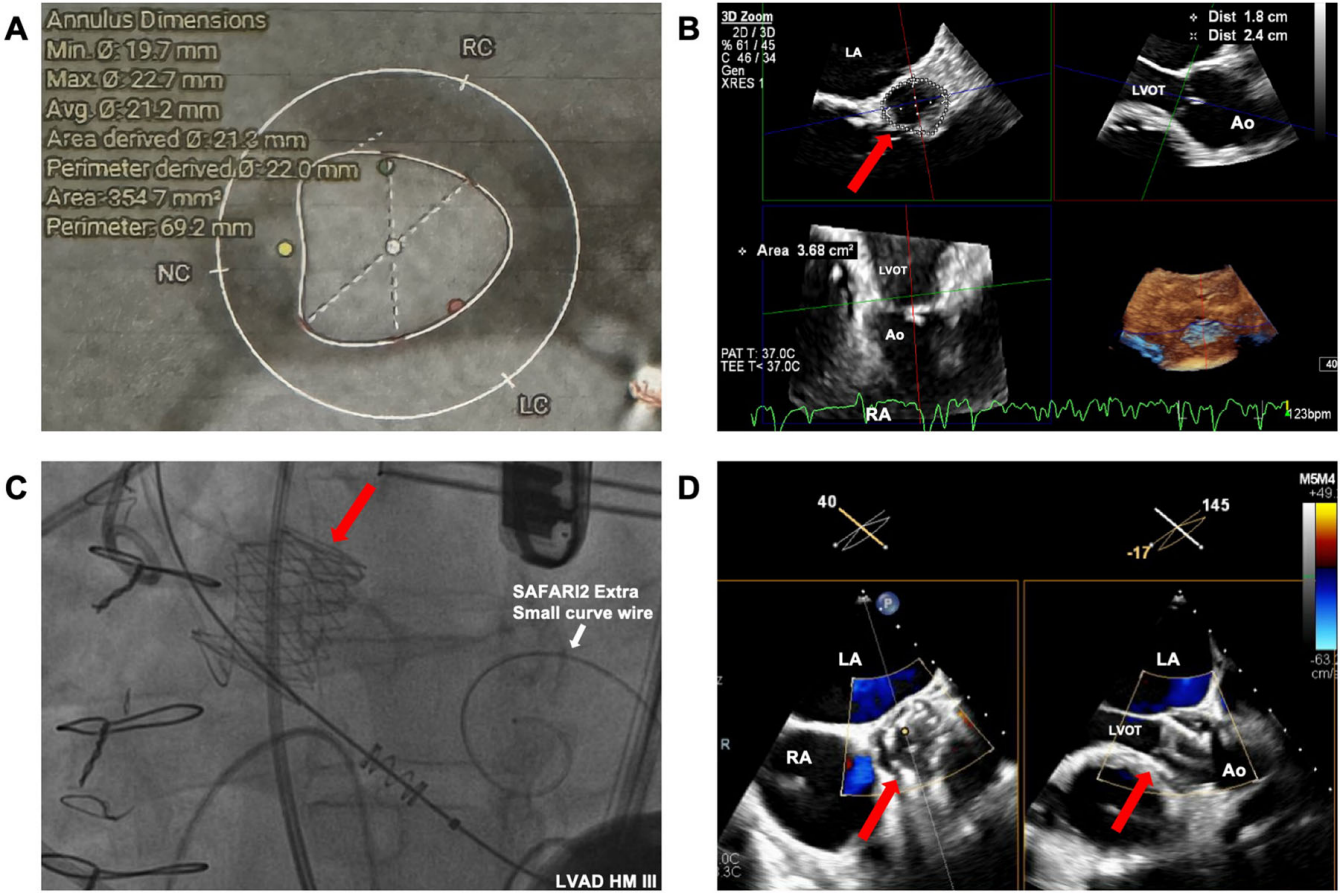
Patient 4. (A) Computed tomography (CT) revealed a small aortic annulus with diameter 1.80 × 2.40 cm, area 368 mm^2^, and perimeter 69.4 mm. (B) Transoesophageal echocardiography (TEE) showing similar measurements [red arrow—aortic annulus]. (C) Successful J‐Valve deployment confirmed on fluoroscopy [red arrow—J‐Valve]. (D) TEE showed no residual AR [red arrow—J‐Valve]. [Color figure can be viewed at wileyonlinelibrary.com]
